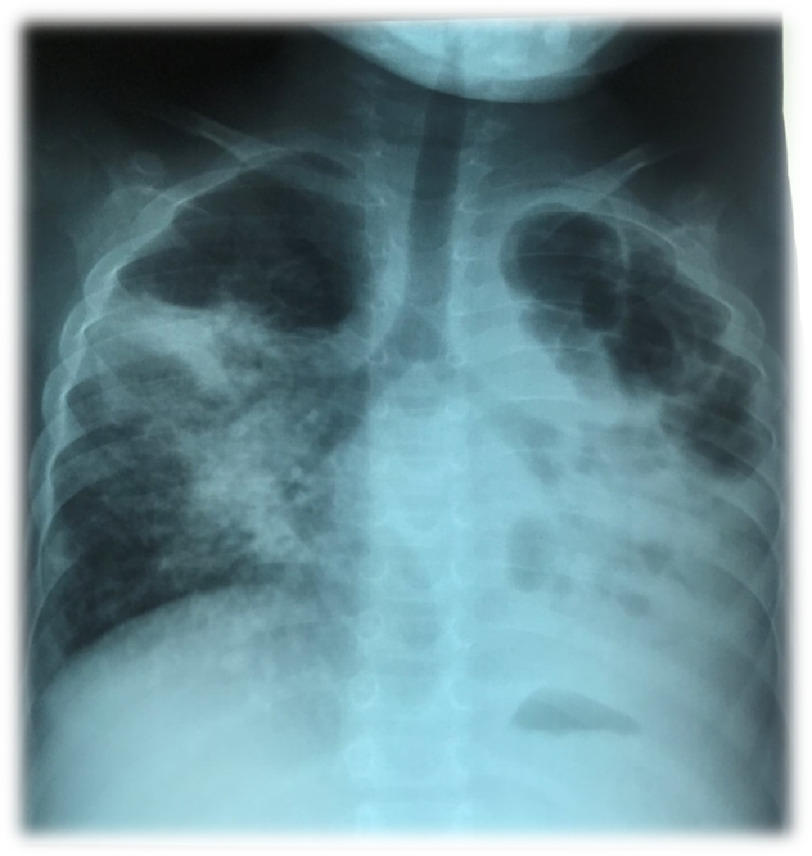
Chest X-ray of a 7-years old girl with perinatal HIV infection, followed by recurrent pulmonary tuberculosis and persistent high viral load associated with poor adherence to therapy for both HIV and tuberculosis. The image shows apical pleural thickening and cardiac silhoute not well defined due to heterogeneous opacities and centrilobular nodular pattern affecting the right medial and basal middle lobe, due to possible transbronchial dissemination from the left lung to the middle lobe of the right lung, suggesting a re-activation of tuberculosis. Left lung “destroyed” as a result of tuberculosis, in the upper lobe there are areas of emphysema and fibrosis, as well as consolidation of the lower lobe with cavitation (cavernous aspect) and bronchiectasis.
